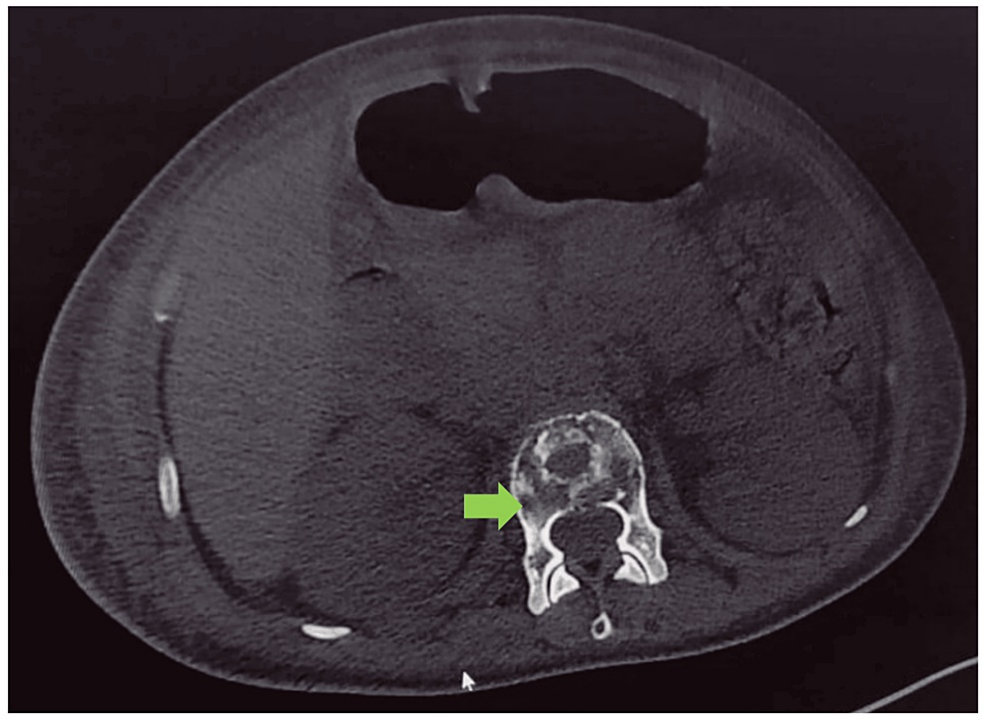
Non-contrast computed tomography showing numerous lytic lesions thoracic vertebral body (green arrow).
